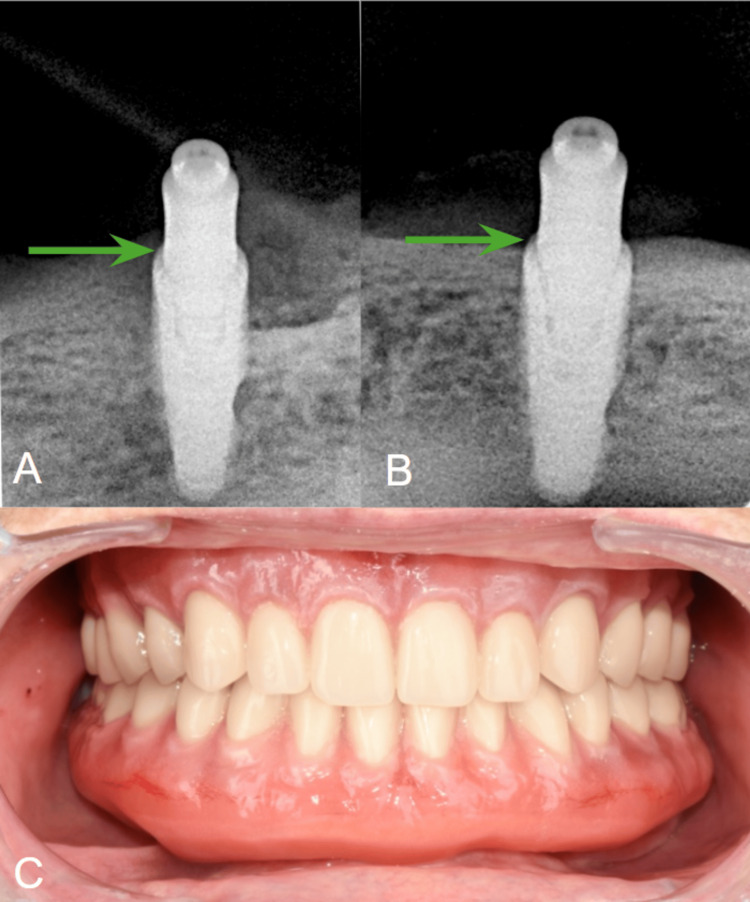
Three-month follow-up after definitive loading. (A) Periapical radiograph of implant 33; (B) periapical radiograph of implant 43 (green arrows); (C) clinical evaluation of the overdenture during the first peri-implant maintenance visit.
